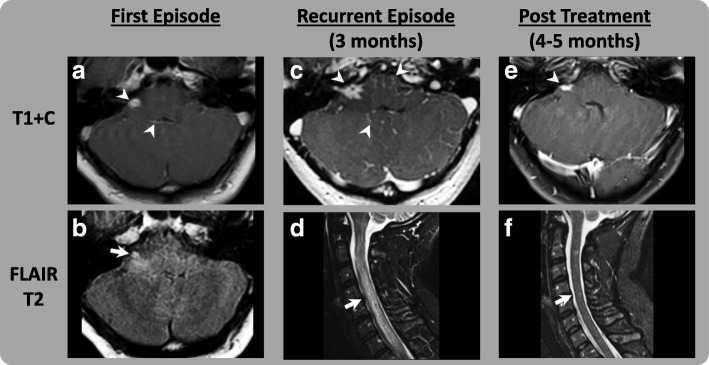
Gadolinium Enhanced MRI Brain and Spine, Images of Patient 1. First Episode (a and b): Abnormal nodular leptomeningeal enhancement, most notably at the R-cerebellar pontine angle (CPA) and 4^th^ ventricle (arrowhead). Lack of FLAIR suppression at the basal cisterns is also most prominent at the R-CPA. There is associated parenchymal edema that extends into the right brachium pontis (arrow). Recurrent Episode (c and d): Worsening leptomeningeal enhancement, prominently involving the R-internal auditory canal (arrowhead). Holocord T2 signal hyperintensity consistent with edema resulting in complete effacement of the CSF space (arrow). Post-treatment (e and f): Mild residual abnormal nodular enhancement at the right IAC (arrowhead) and near complete normalization of the cord diameter. There is only trace residual abnormal signal (arrow)
